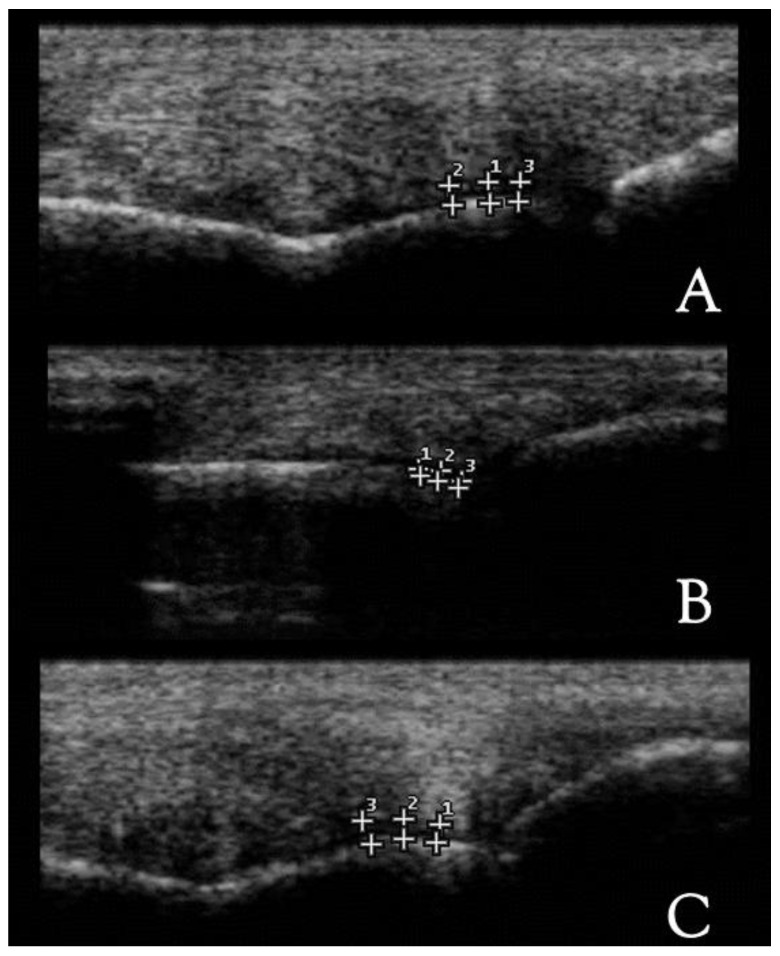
Longitudinal ultrasound views from a thoroughbred MCP joint. (A) Dorsal, (B) dorso-lateral and (C) dorso-medial scans; (1, 2, 3) cartilage thickness consecutive measurements. Proximal is to the left. B-mode, linear probe, 7.5 MHz.
